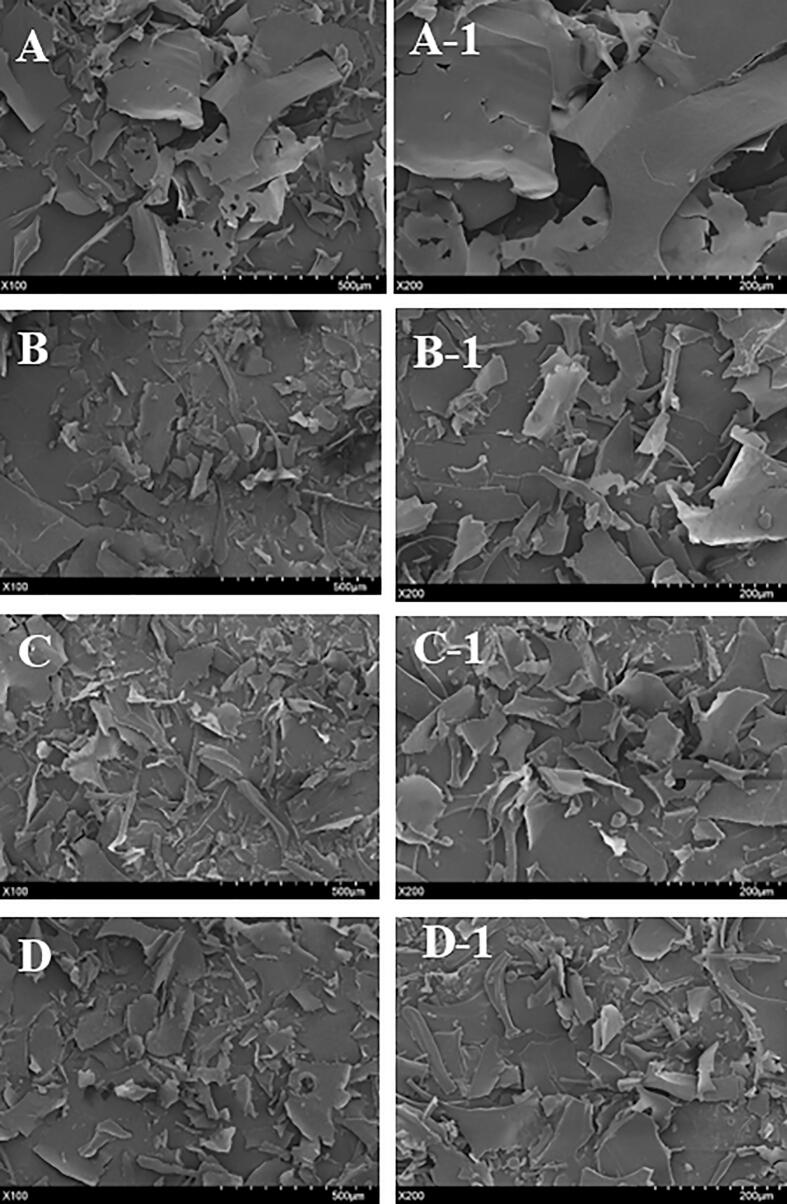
SEM image of HCP and ultrasonic degraded polysaccharide A: (magnification 100x, scale bar 500 µm) of HCP. A-1: (magnification 200x, scale bar 200 µm) of HCP. B: (magnification 100x, scale bar 500 µm) of U200. B-1: (magnification 200x, scale bar 200 µm) of U200. C: (magnification 100x, scale bar 500 µm) of U400. C-1: (magnification 200x, scale bar 200 µm) of U400. D: (magnification 100x, scale bar 500 µm) of U600. D-1: (magnification 200x, scale bar 200 µm) of U600.
